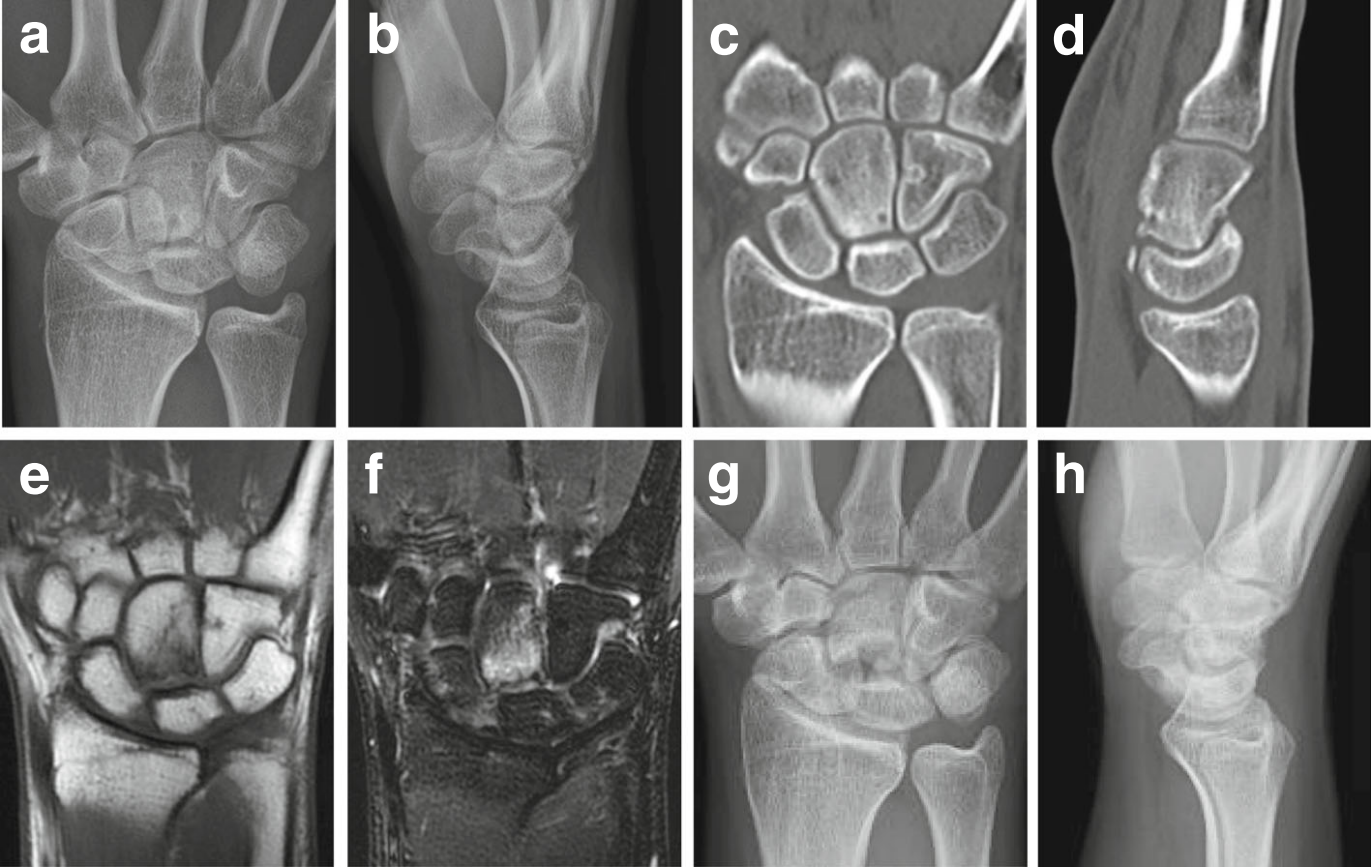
AP radiograph, CT, and MRI findings. a, b The preoperative radiographs showed collapse of the proximal portion of the capitate along with osteonecrosis. c, d The preoperative CT scans showed collapse of the proximal portion of the capitate along with osteonecrosis, a bone cyst in the proximal capitate, and a free body in the palmar proximal portion of the capitate. e A preoperative coronal T1-weighted MRI image showed low signal intensity at the proximal capitate. f A preoperative coronal STIR MRI image showed high signal intensity at the proximal capitate. g, h At the 2-year follow-up after surgery, the radiographs showed no further collapse of the capitate or progression of carpal instability
